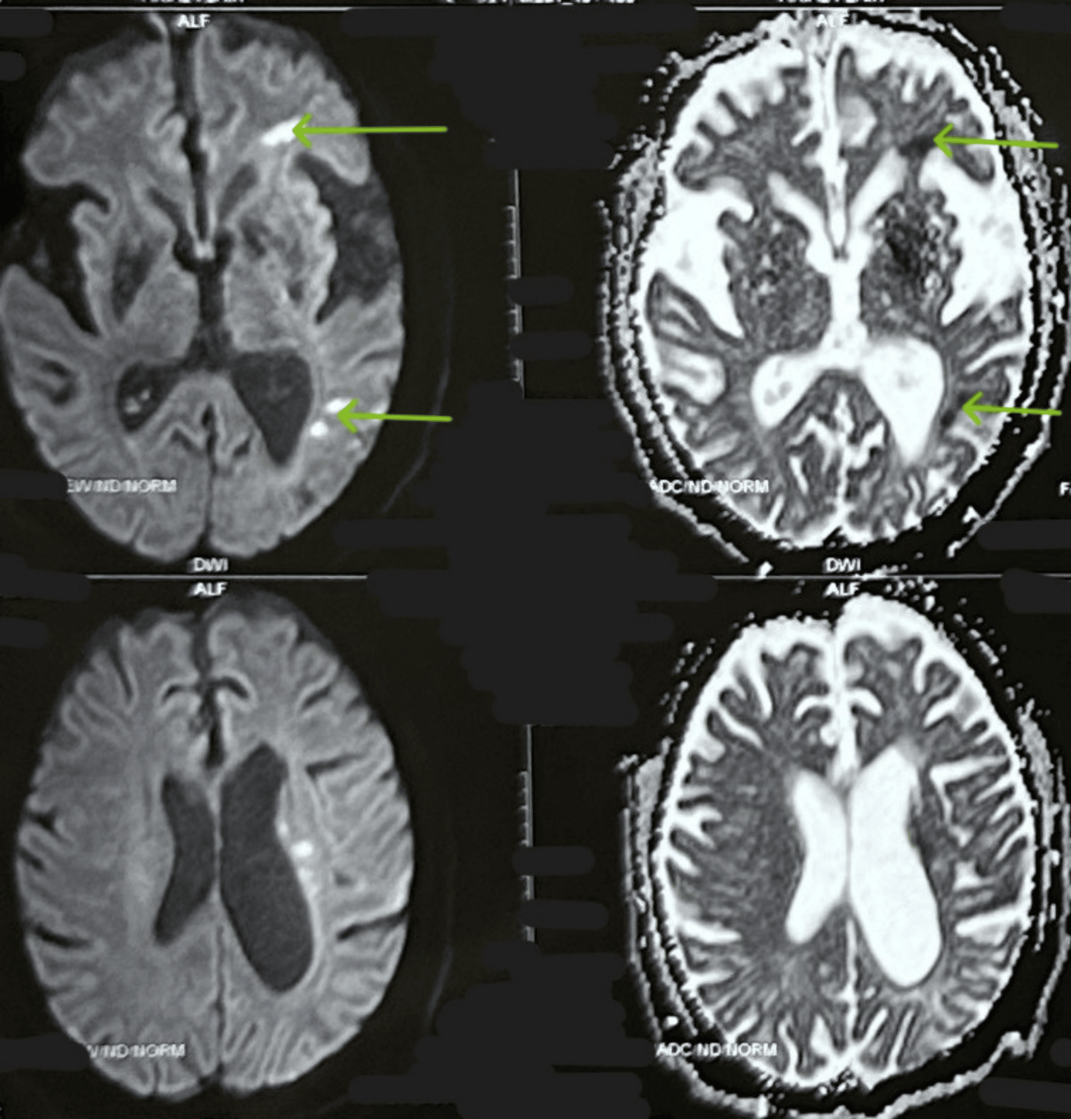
Diffusion-weighted imaging (DWI) with corresponding apparent diffusion coefficient (ADC) maps showing acute lacunar infarcts in the left frontal and occipital lobes The green arrows denote the areas of lacunar infarcts in the left frontal and occipital lobes.
